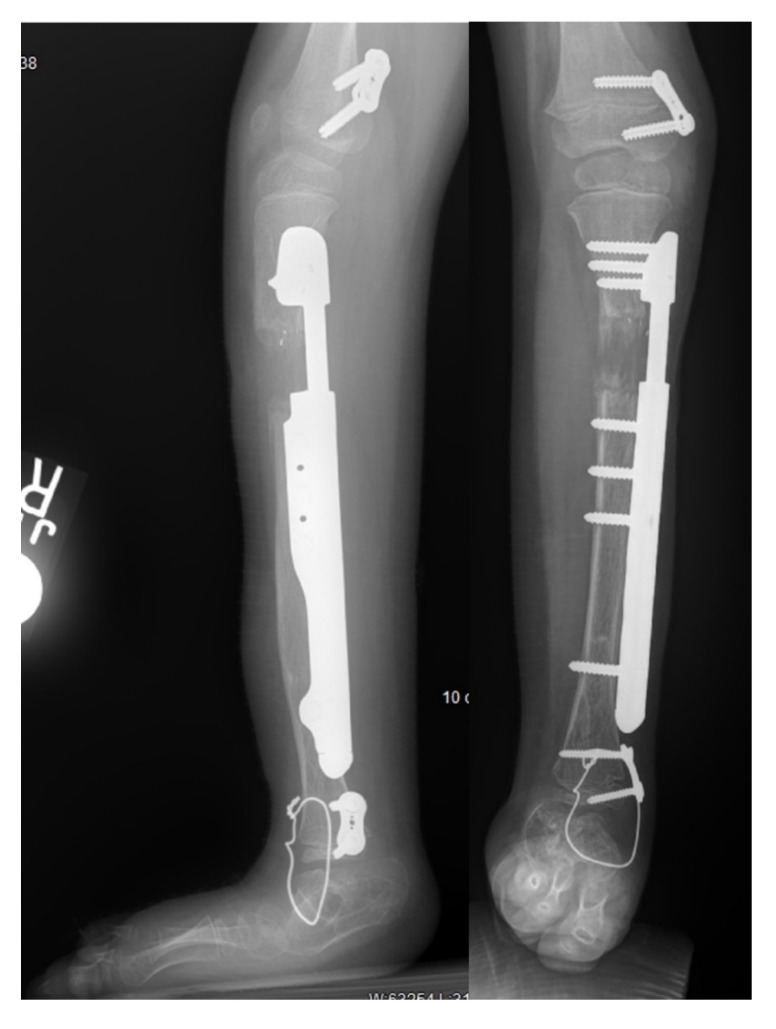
AP and lateral radiographs of a 4-year-old girl who had a previous SUPERankle procedure and one 5 cm lengthening with an external fixator. She had a Precice plate applied to the medial side of the tibia. To prevent equinus the foot was tethered anteriorly with a temporary arthrodesis wire. The Precice plate (Nuvasive Specialized Orthopedics, Aliso Viejo, CA, USA) can lengthen up to 4.5 cm.
